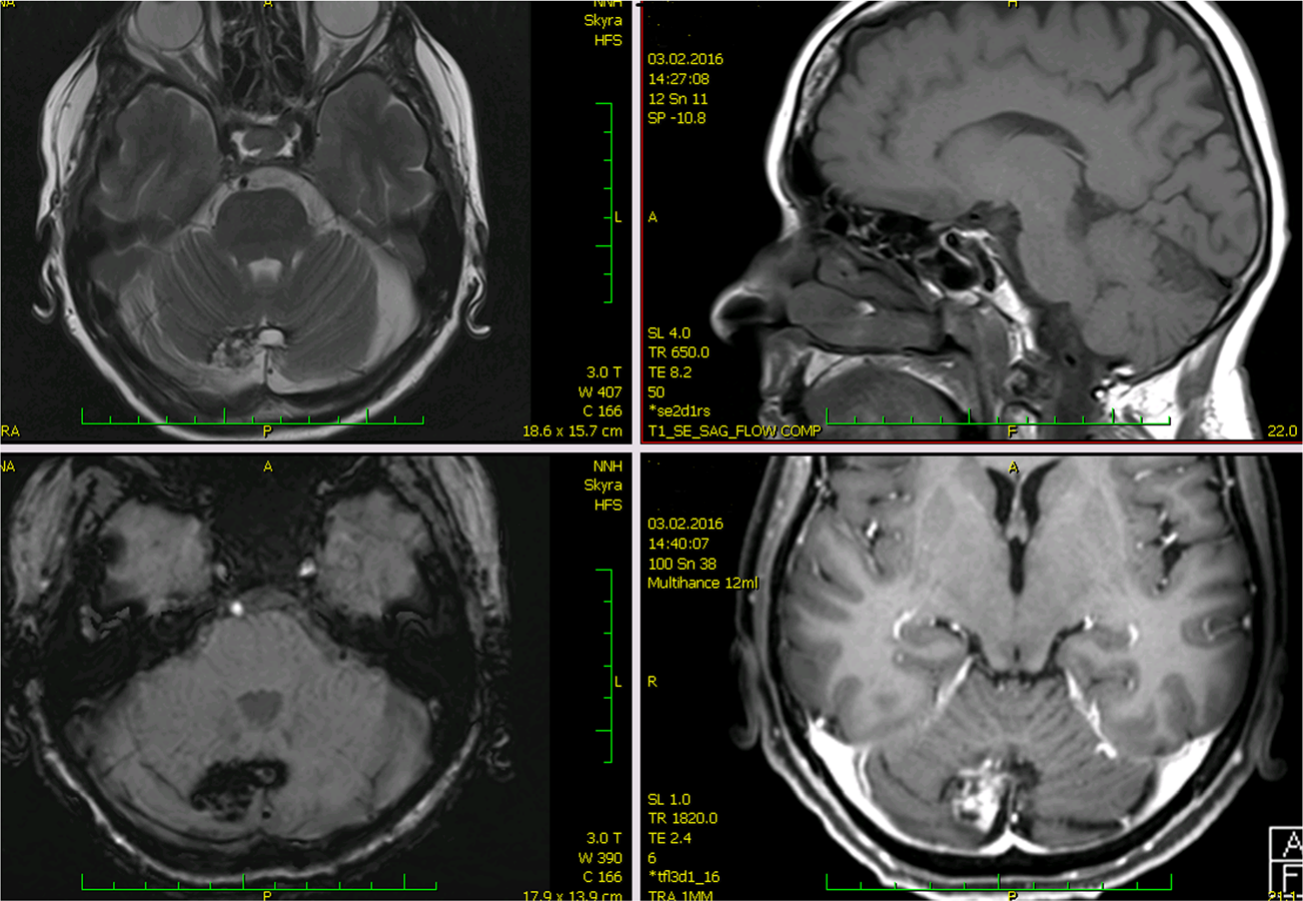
MRI appearance of the cavernous malformation-like lesion. AVM was irradiated 18 years prior; at the time of irradiation, the AVM nidus was 0.71 cc, AVM obliteration was proven 3 years later. There were no signs of hemorrhage. Recent MRI showed extended hemosiderin deposition and post-gadolinium enhancement covering a larger area than the AVM. (*Upper left*: TSE T2 transversal scan,* upper right*: SE T1 sagittal scan,* lower left*: T2 SWI 3D transversal scan,* lower right*: GE T1 3D post-gadolinium transversal scan)
